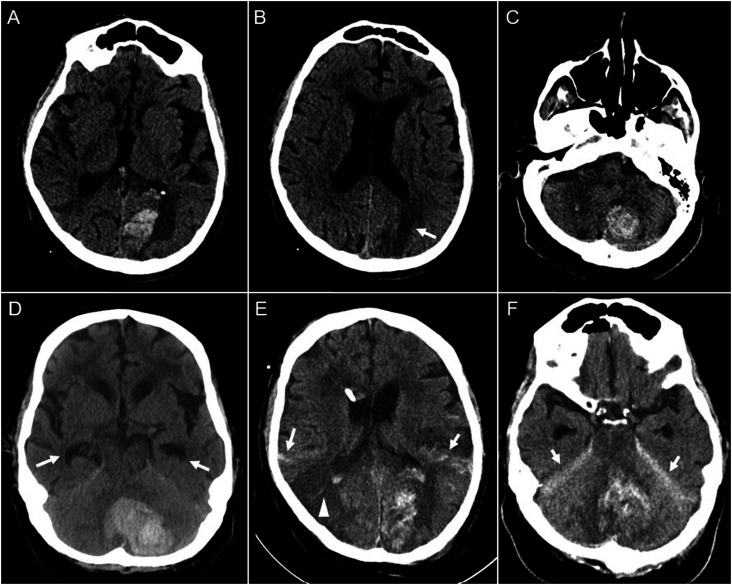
Serial CT head images after representing three weeks later. Initial CT head notable for hemorrhagic conversion of subacute left PCA stroke (A), new left parietal infarct (B, arrow), and left greater than right bilateral cerebellar hemorrhages (C). Repeat CT head obtained after clinical deterioration shows further expansion of the left cerebellar hemorrhage (D) with associated hydrocephalus as evidenced by enlargement of temporal horns (arrows). CT head after sub-occipital craniectomy and placement of EVD shows new right temporal-occipital ischemic stroke (E, arrowhead) as well as bilateral subarachnoid hemorrhage (E, arrows) and tentorial subdural hematomas (F, arrows).
